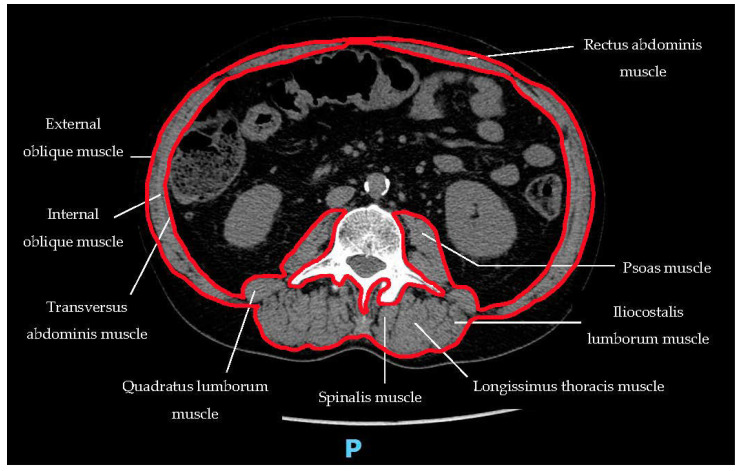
Example of delineation of the skeletal muscle area on a CT scan at the level of the third lumbar vertebra.
